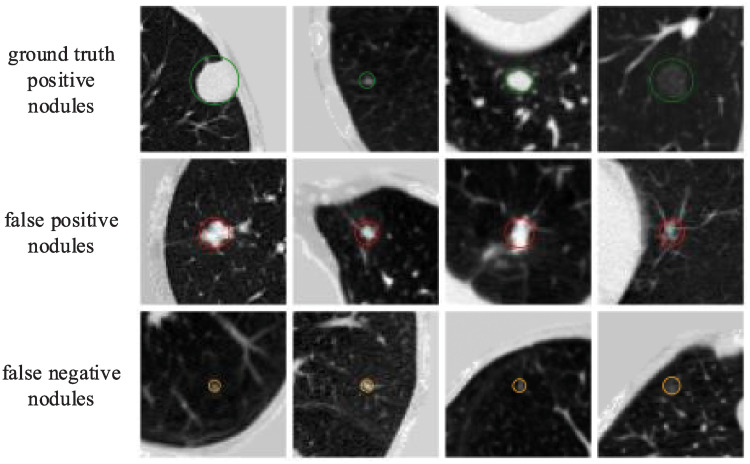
Experimental results. The CT images in Fig 6 were accessed through the links available on this page: https://luna16.grand-challenge.org/Download/ and had not been previously published and/or copyrighted.
